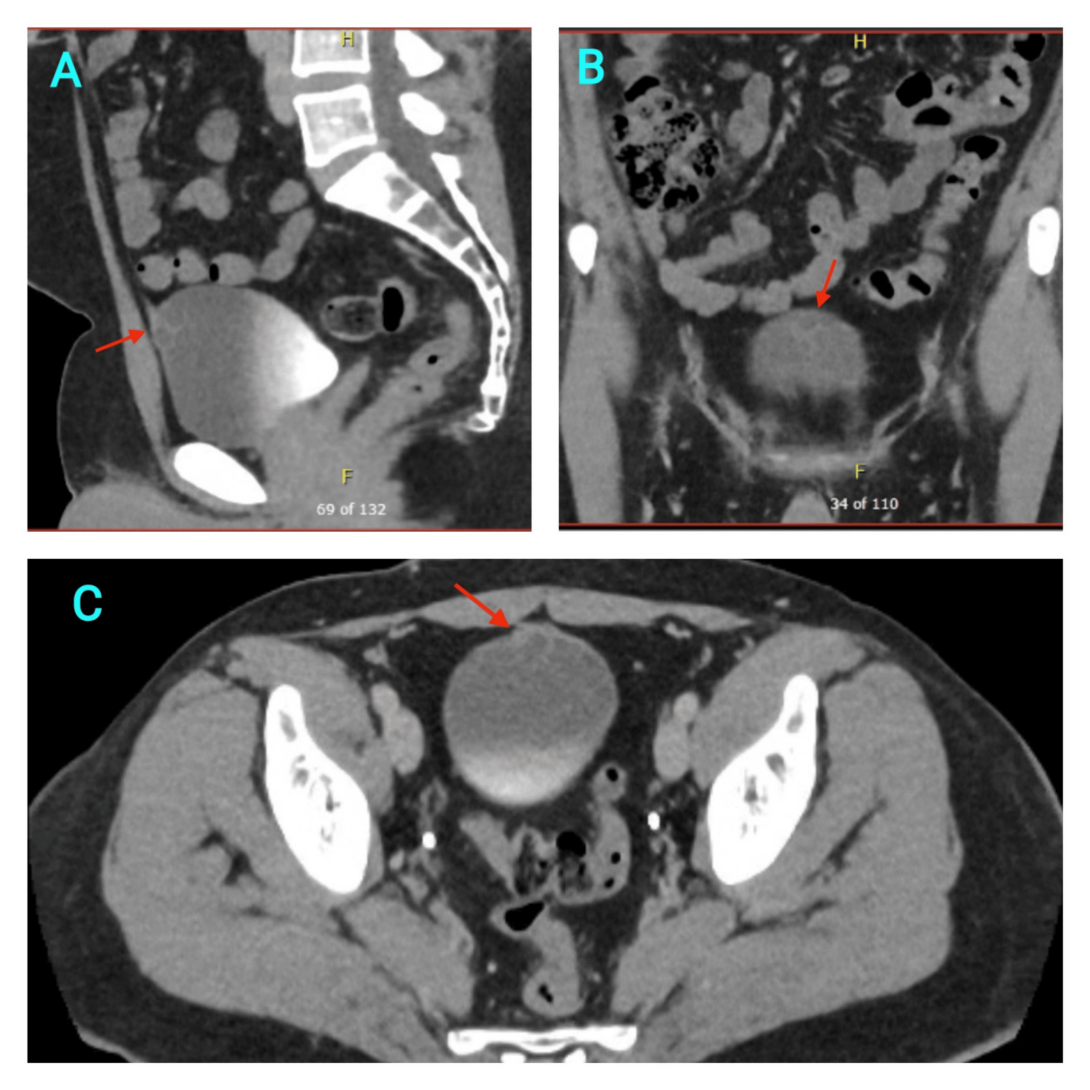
Contrast-enhanced CT scan of the abdomen and pelvis with a delayed urographic phase showing the urachal cyst (red arrows) in three different planes including sagittal (A), coronal (B) and axial (C) planes.
